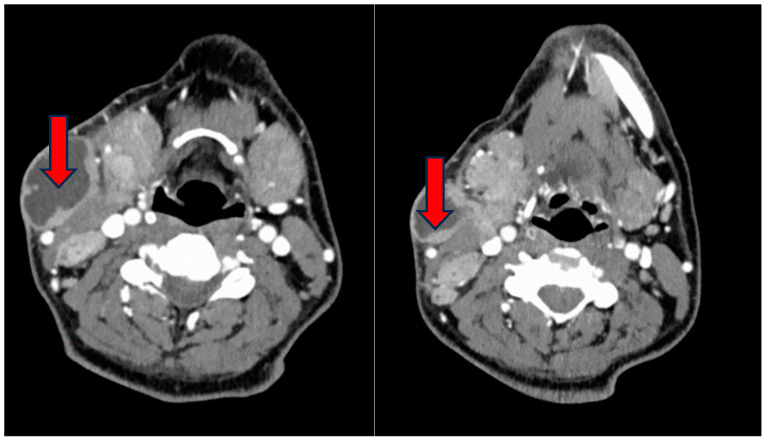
Neck CT scan confirming a conglomerated centrally colliquated mass (red arrows), extending anteriorly and posteriorly to the sternocleidomastoid muscle of 8 × 4.4 × 10 cm.
